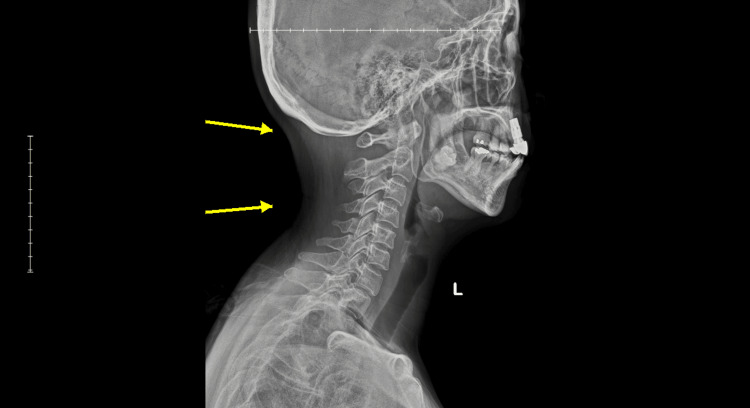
Lateral cervical radiograph demonstrating loss of normal cervical lordosis and straightening of the cervical spine. Arrows indicate the cervical spine, highlighting the absence of normal lordotic curve.
